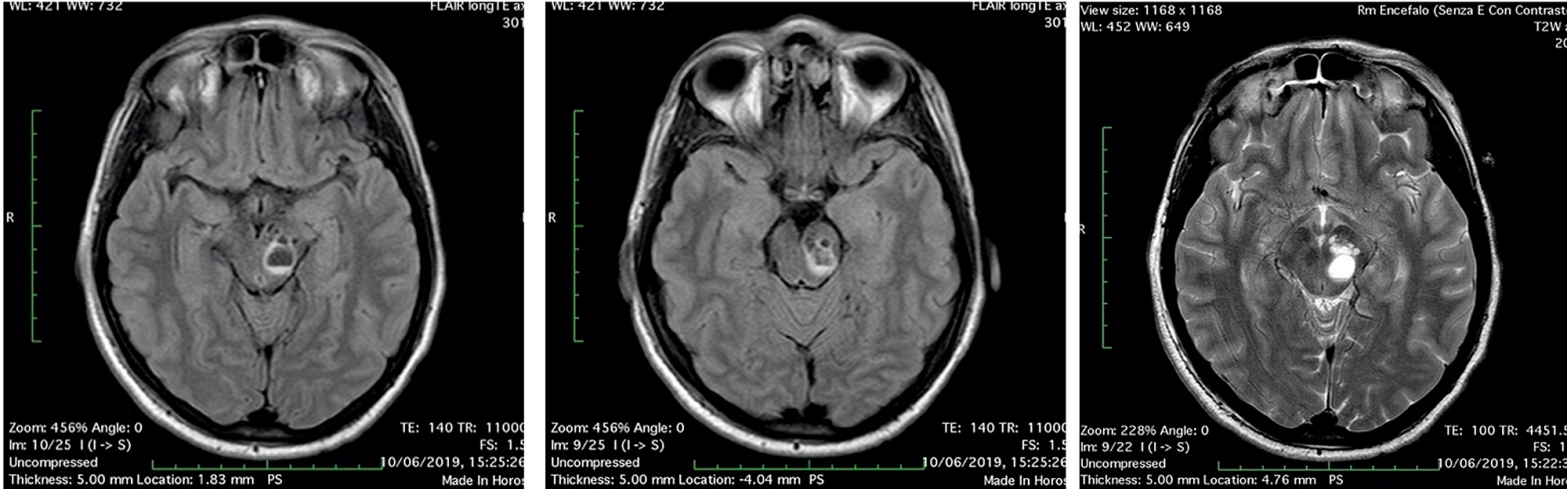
Head MRI scan “the day after”: the multiloculated cyst in the left midbrain showed fluid-fluid level, with enlargement of left cerebral peduncle and a slight mass effect, referring to enlargement and bleeding of PVSs (3A-3B: FLAIR axial image; 3C: T2 axial image). PVSs, perivascular spaces
